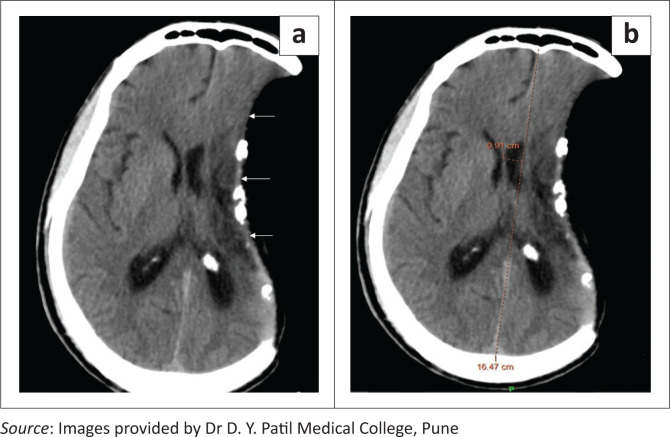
Paradoxical herniation. Axial soft tissue window CT brain images (a, b) reveal a left fronto-temporo-parietal craniectomy with a sunken skin flap (arrows), deformation of the underlying brain parenchyma, midline shift of 9.1 mm (b) and subfalcine herniation to the right side. Associated meningogaleal complex calcifications are also seen.
